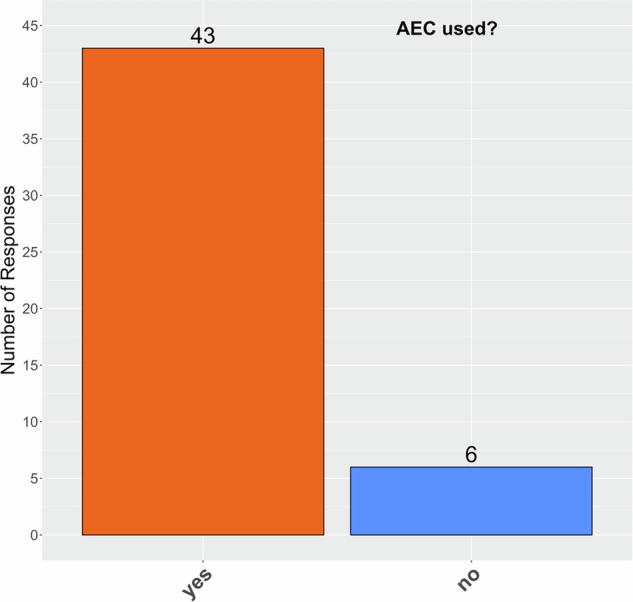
Declared use of automatic exposure control (AEC) in CT image acquisition protocols for lung cancer screening
